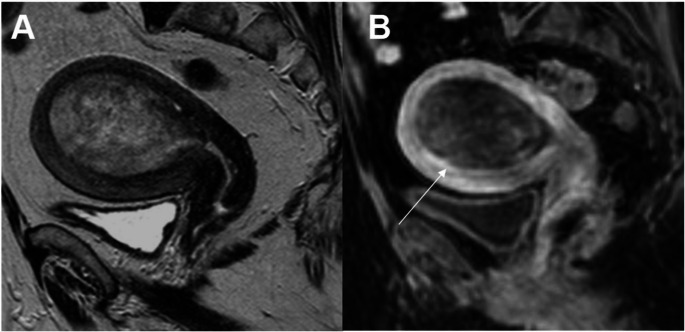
Stage IC carcinosarcoma, no LVSI in a 67-year-old woman. A Sagittal T2-weighted fast spin-echo (FSE) image (TR/TE: 4500/100 ms; slice thickness: 3 mm) and B Sagittal contrast-enhanced 3D T1-weighted fat-suppressed LAVA sequence (TR/TE: 4.2/2.1 ms; slice thickness: 2 mm; post-gadolinium, delayed phase at 3 min) show a bulky endometrial tumor distending the endometrial cavity without myometrial invasion. Preservation of the junctional zone is better appreciated on dynamic images after contrast (arrow). Pathology confirmed carcinosarcoma limited to the endometrium
